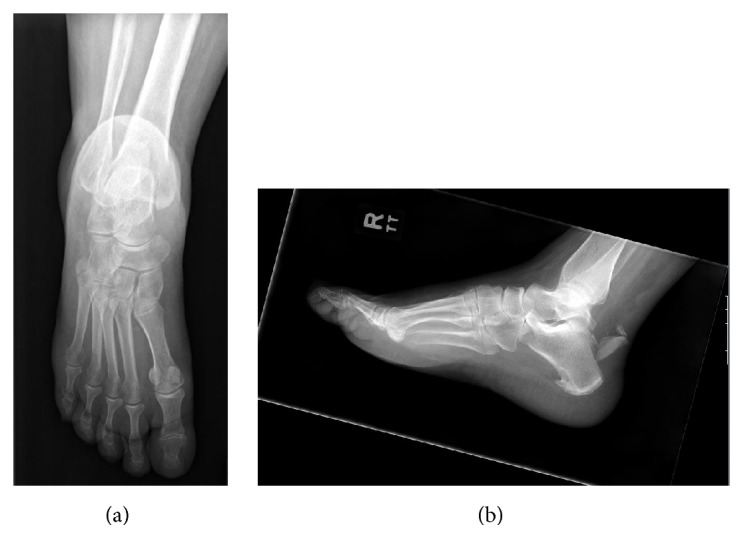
Anteroposterior (a) and lateral (b) radiographs demonstrating displaced fragmented calcaneal tuberosity avulsion following injury.
